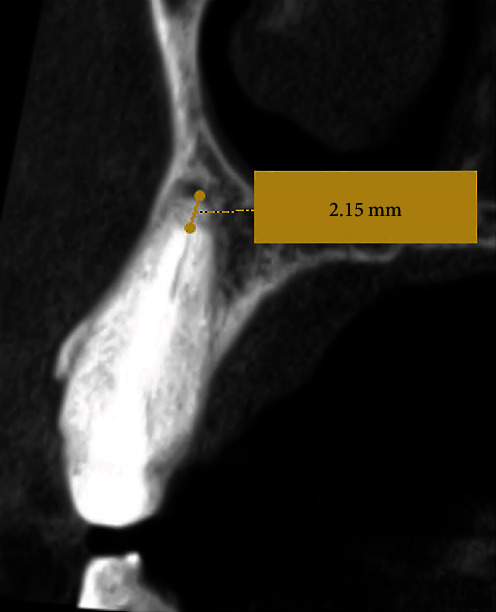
Preoperative CBCT assessment. Sagittal slice of tooth #23 confirms apical root resorption with the radiolucent area, 2.15 mm short obturation, and fiber post cemented up to the middle third.
